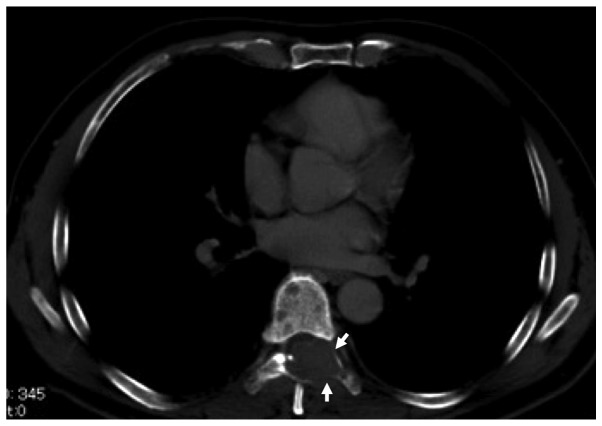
Pretreatment computed tomography revealed a metastatic bone tumor in the ninth thoracic vertebral arch.
